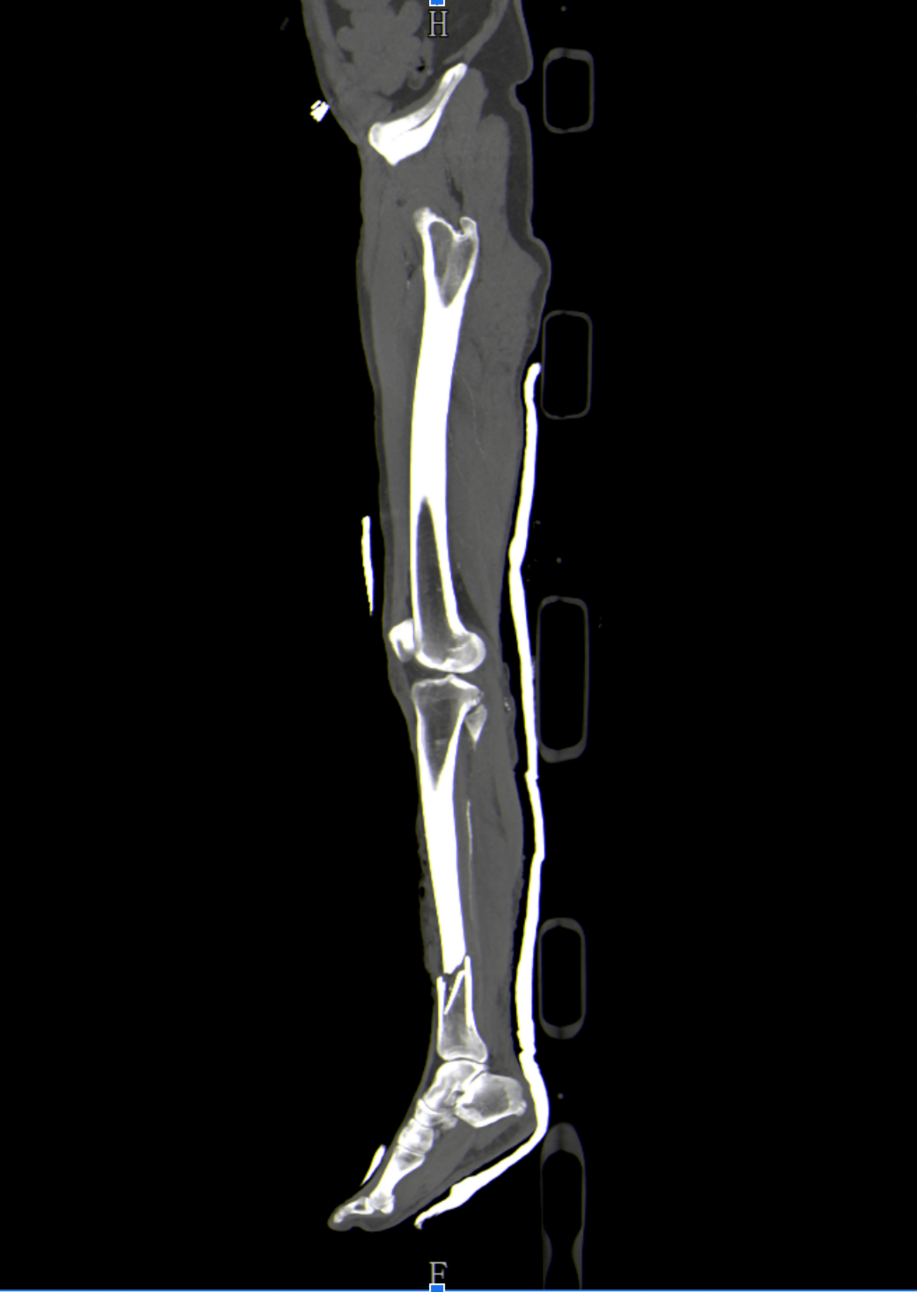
CT scan confirming left lower limb fracture. F: foot; H: head.
